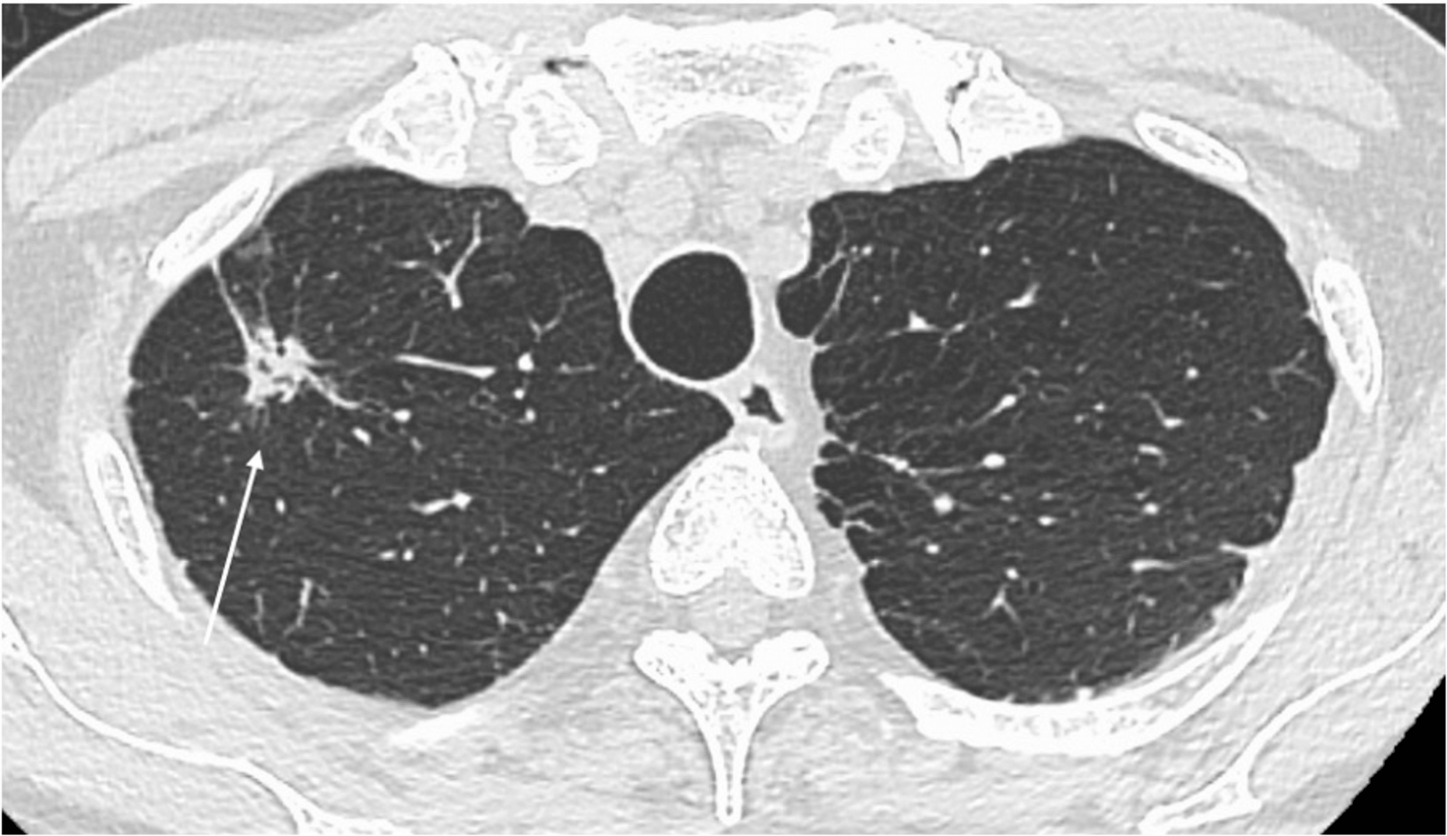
Chest computed tomography (CT) findings (axial). An irregular nodule 15 x 14 mm in size was seen in the S1 upper lobe of the right lung (arrow).
